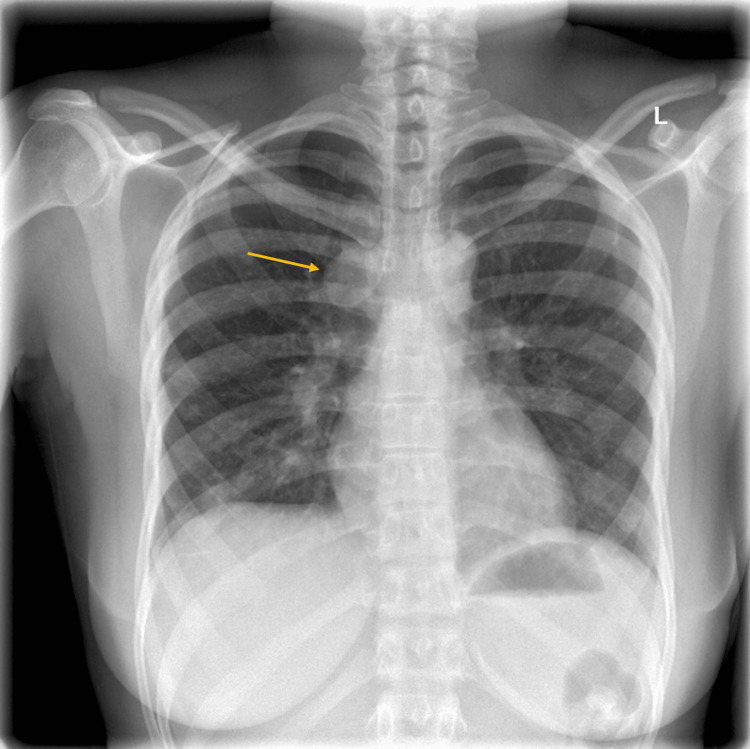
A posterolateral view of a chest X-ray. The image demonstrates an oval-shaped high-density focus measuring 2.9 × 2 cm and projecting over the medial aspect of the left upper lung overlying the fifth and sixth posterior ribs (yellow arrow).
